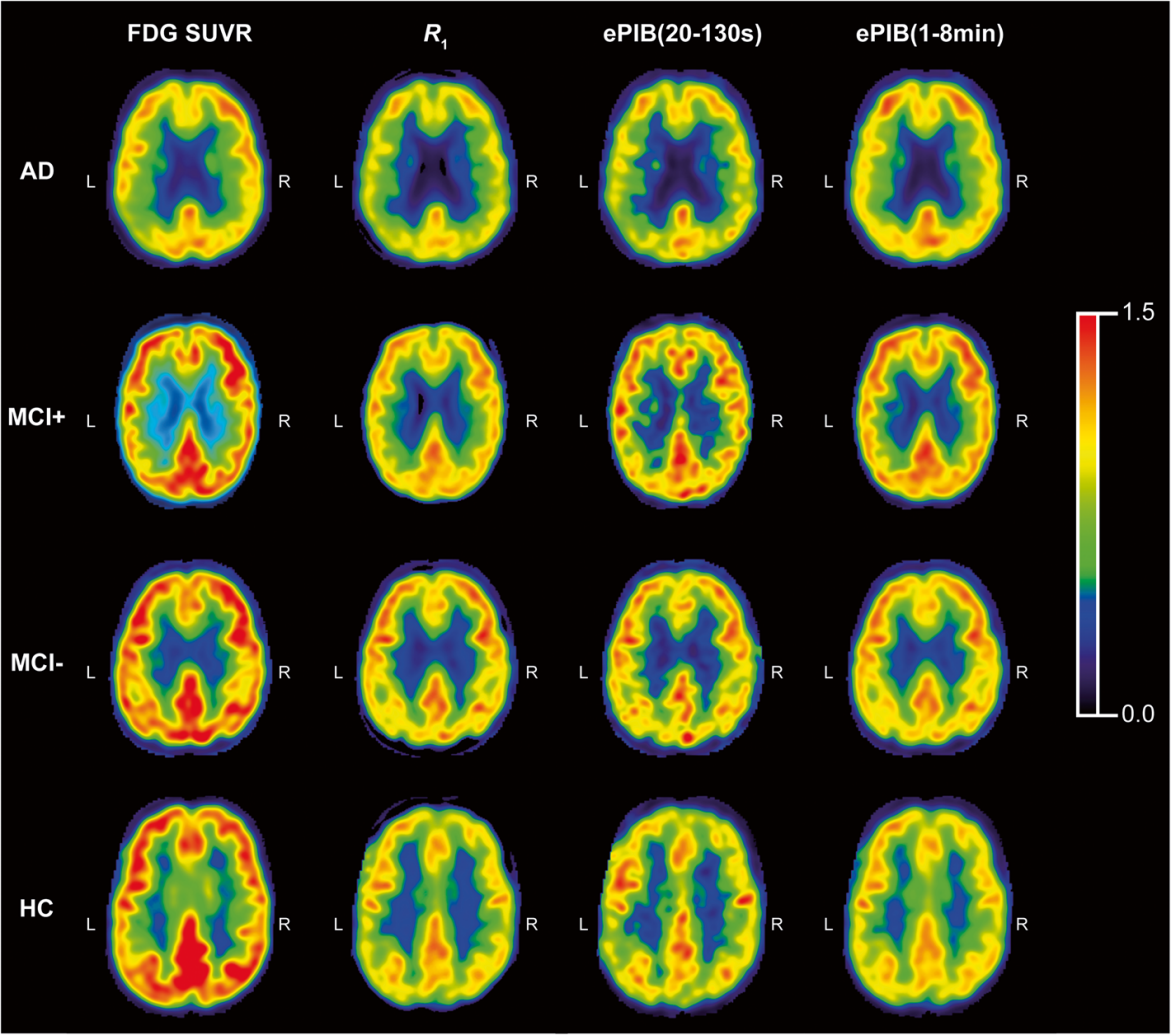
Representative studies. Transaxial slices of the brain are shown. From left to right: FDG SUVR images, *R*_1_ parametric maps, ePIB (20–130 s), and ePIB (1–8 min) images. On the first row, images from an AD patient; on the second, an MCI+ subject; on the third, an MCI− subject; and at the bottom, an HC subject. All colour scales are adjusted to the same range
